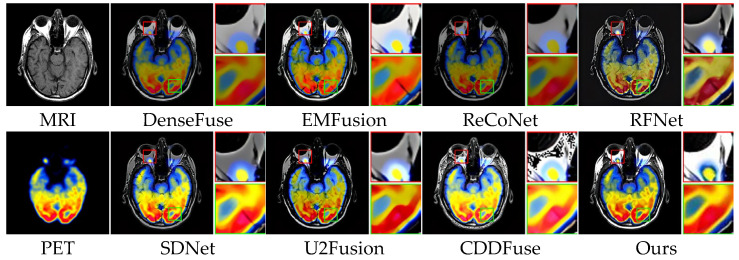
Visual comparison for “MRI-PET-16” in MRI-PET MIF.
